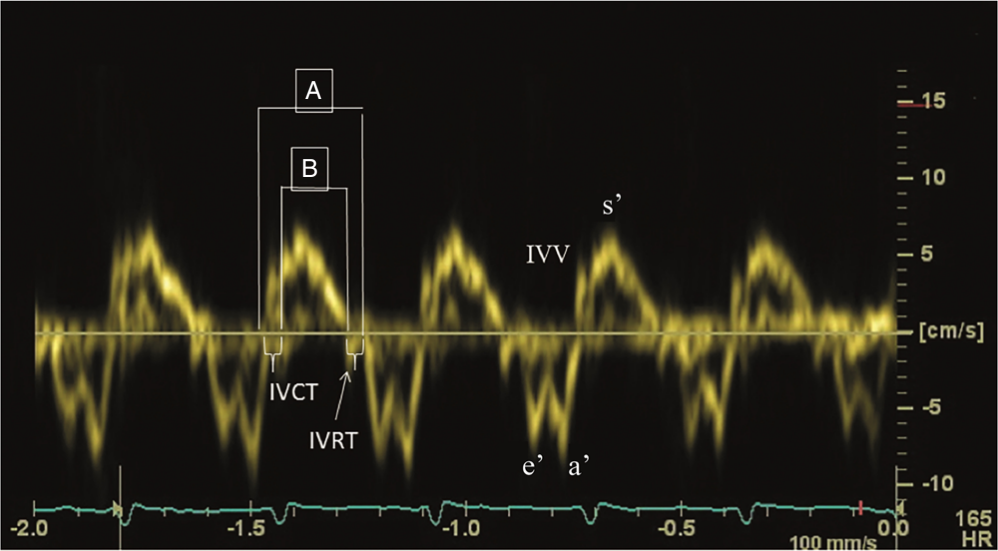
Myocardial Performance Index—MPI or Tei index. Pulse wave Tissue Doppler waveform (isovolumic contraction time (IVCT), isovolumic relaxation time (IVRT), peak isovolumic systolic (IVV), early diastolic (e′), late diastolic (a′), peak systolic (s′) velocity). Myocardial performance index = the sum of isovolumic contraction and relaxation time divided by ejection time (*A*−*B*/*A*)
